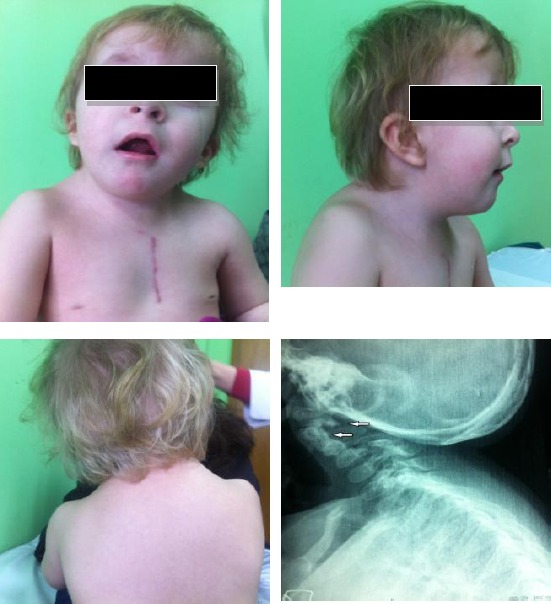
Case 2. Patient with Klippel-Feil syndrome and anomaly of the occipito-cervical junction. The images show an elevated left shoulder due to a Sprengel anomaly, a short, webbed neck, and a low hairline. (Scar on the thorax after surgical repair of nonrestrictive atrial septal defect). X-ray shows occipito-cervical junction*.
